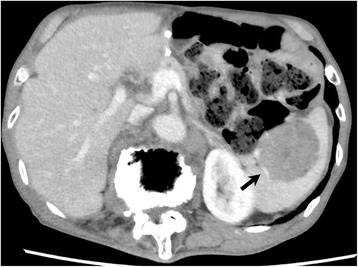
Abdominal computed tomography showing a well-defined mass in the spleen measuring 4 cm in diameter (*arrow*)
